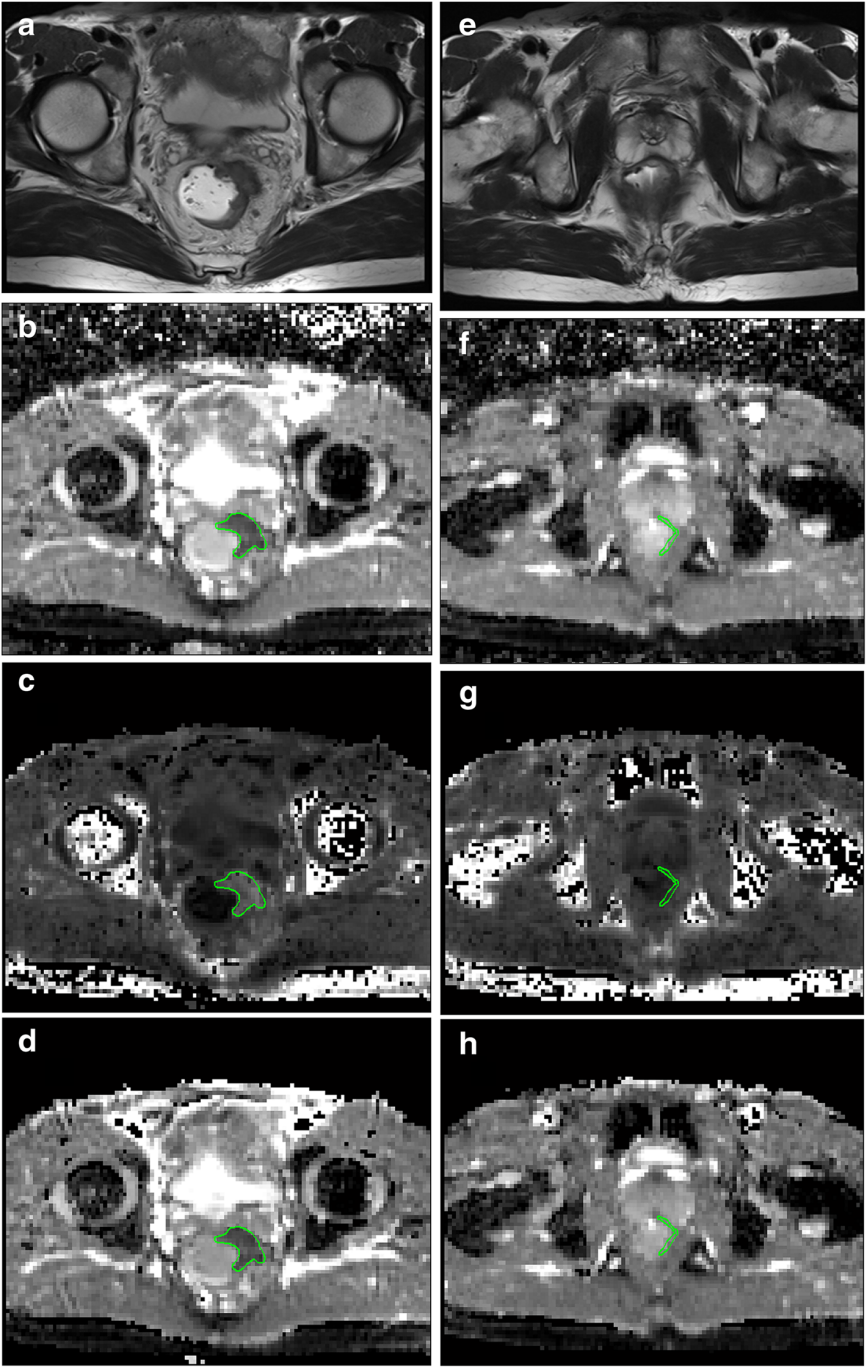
Images above show Grade 1 AC and unaffected rectal wall in a 62-year-old man. Axial T2-weighted MR image shows the AC along the left wall of the rectum (a). ADC map shows low-signal-intensity tumor (ADC = 1.09 ± 0.24 × 10^− 3^ mm^2^/s, b). MK map shows high-signal-intensity tumor (MK = 1.00 ± 0.19, c). MD map shows low-signal-intensity tumor (MD = 1.26 ± 0.24 × 10^− 3^ mm^2^/s, d). Axial T2-weighted MR image shows the unaffected rectal wall (e). ADC map shows high-signal-intensity rectal wall (ADC = 1.69 ± 0.10 × 18^− 3^ mm^2^/s, f). MK map shows low-signal-intensity rectal wall (MK = 0.57 ± 0.10, g). MD map shows high-signal-intensity rectal wall (MD = 2.04 ± 0.21 × 10^− 3^ mm^2^/s, h)
